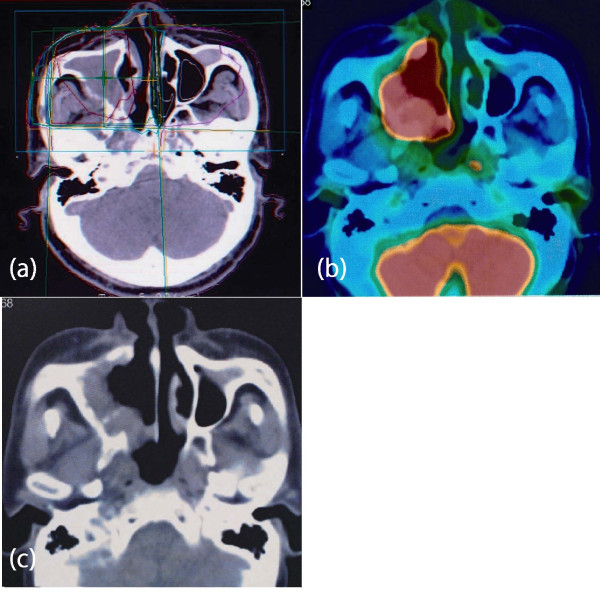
(a) Dose distribution of RT for NHL of the maxillary sinus. (b) and (c) PET-CT showing second SCC infiltrating the bone.
